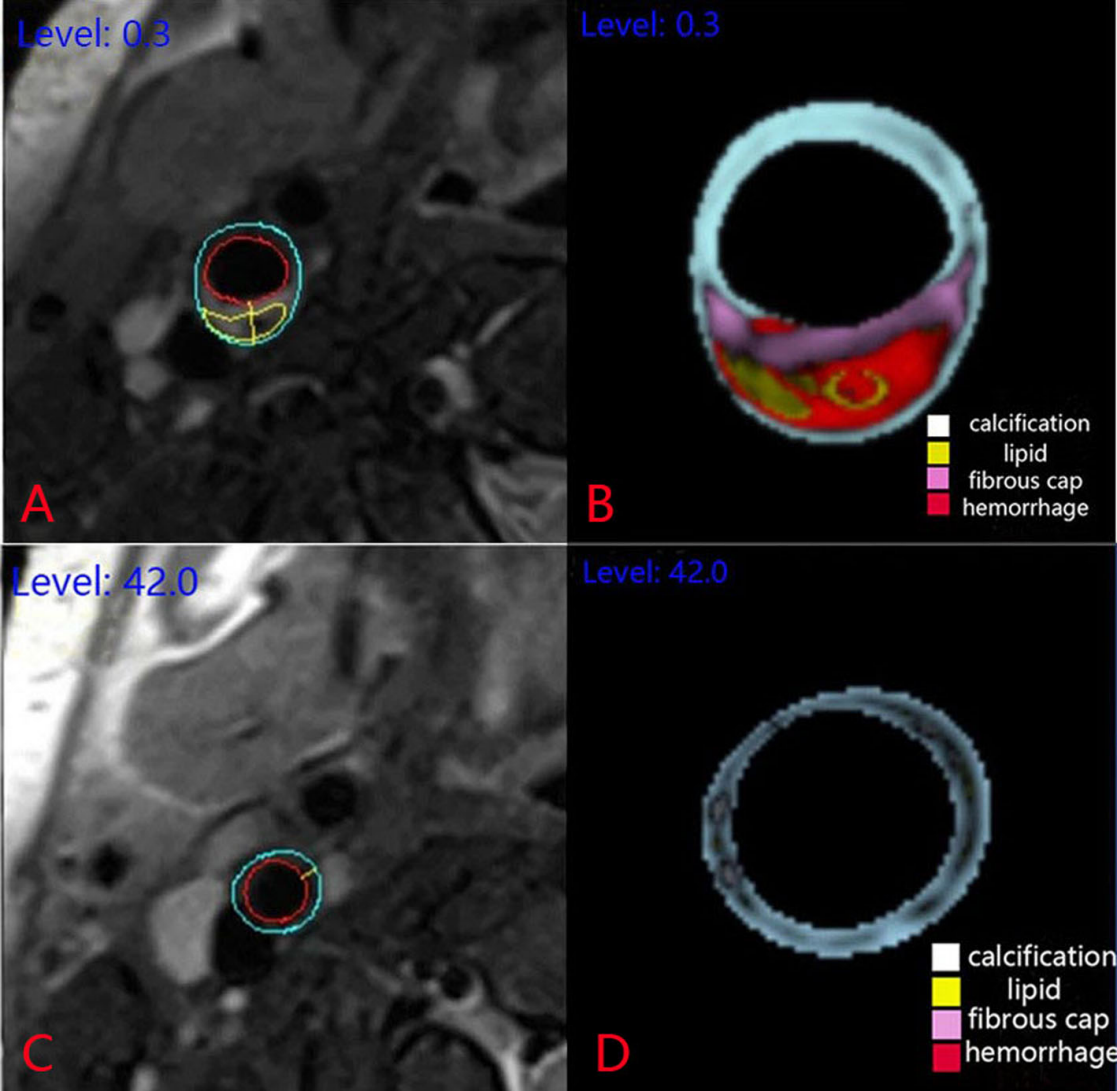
a and b: Before antiplatelet therapy, the right internal carotid artery was selected as the target lumen, and at the same level, the vessel wall magnetic resonance imaging showed a large number of thrombus and lipid core can be seen in the wall of the tube, it means that the mural thrombus is unstable plaque. c and d: After antiplatelet therapy, the right internal carotid artery was selected as the target lumen, and at the same level, the vessel wall magnetic resonance imaging showed the disappearance of abnormal components in the lumen
